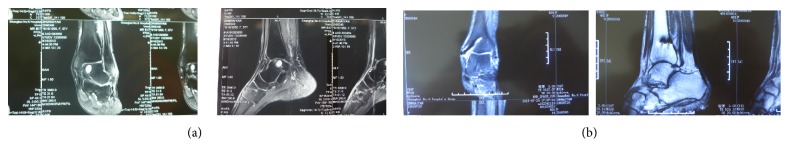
The MRI manifested that the large size of subchondral cystic lesion (a) was successfully repaired with a congruent curvature (b).
